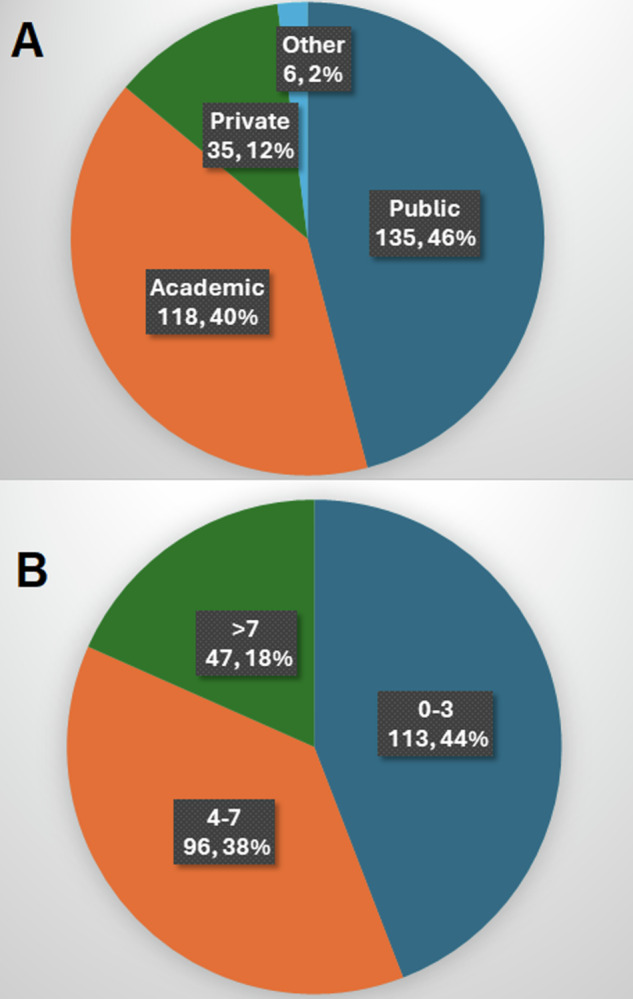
Pie charts indicate (A) the number and proportion of total respondents (*n* = 294) based on public institutions, academic institutions and private practice institutions. Since there could be overlap in some of these categories where an institution could be both public and academic, the respondents were asked to pick the best possible single option based on their work profiles. B The number of post-certification radiologists regularly reporting head and neck MRI in each institution (0–3, 4–7, and > 7)
